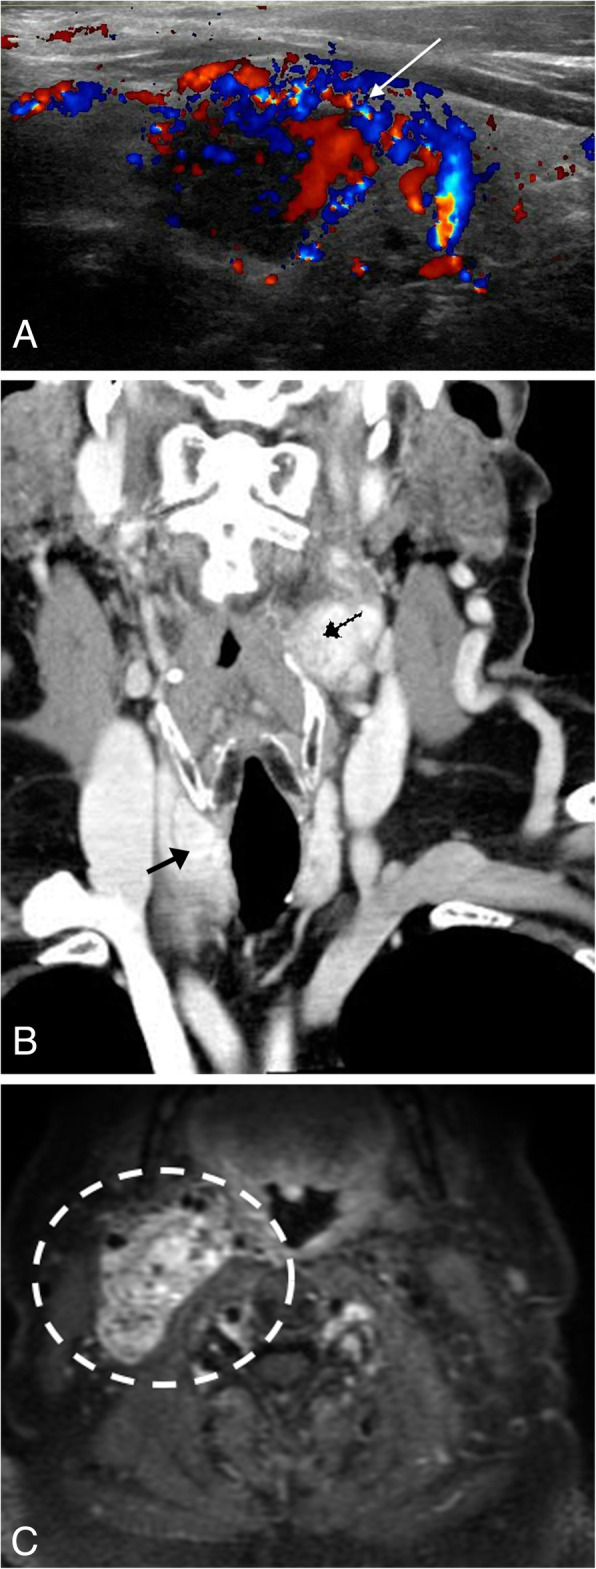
Paraganglioma. a Ultrasound Doppler image shows a hypervascular (arrow) lesion within the right lobe of the thyroid. b Coronal CT image post contrast demonstrates an enhancing lesion within the medial aspect of the right lobe of the thyroid (solid arrow) and in the left carotid space (dashed arrow), both of which were biopsied and shown to be paragangliomas. c Axial T2WI MR of the neck base in a companion case demonstrates the classic salt and pepper appearance of the lesion. The high T2 signal (salt) corresponds to the areas of hemorrhage, and the low signal (pepper) corresponds to the flow voids within the lesion
